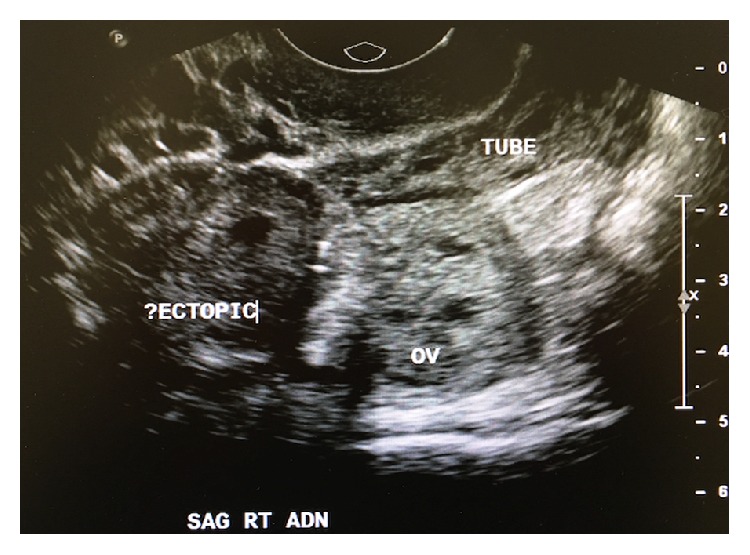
Transvaginal ultrasound of right adnexa.
